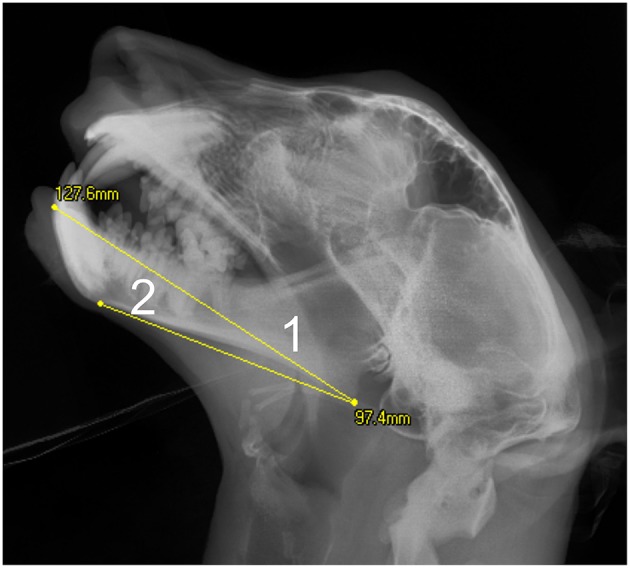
Extent of measurements on the mandible taken from the lateral skull radiographs: 1- Mandibular length1; 2- Mandibular length2. On this cropped image the 100 mm scale bar is not visible.
